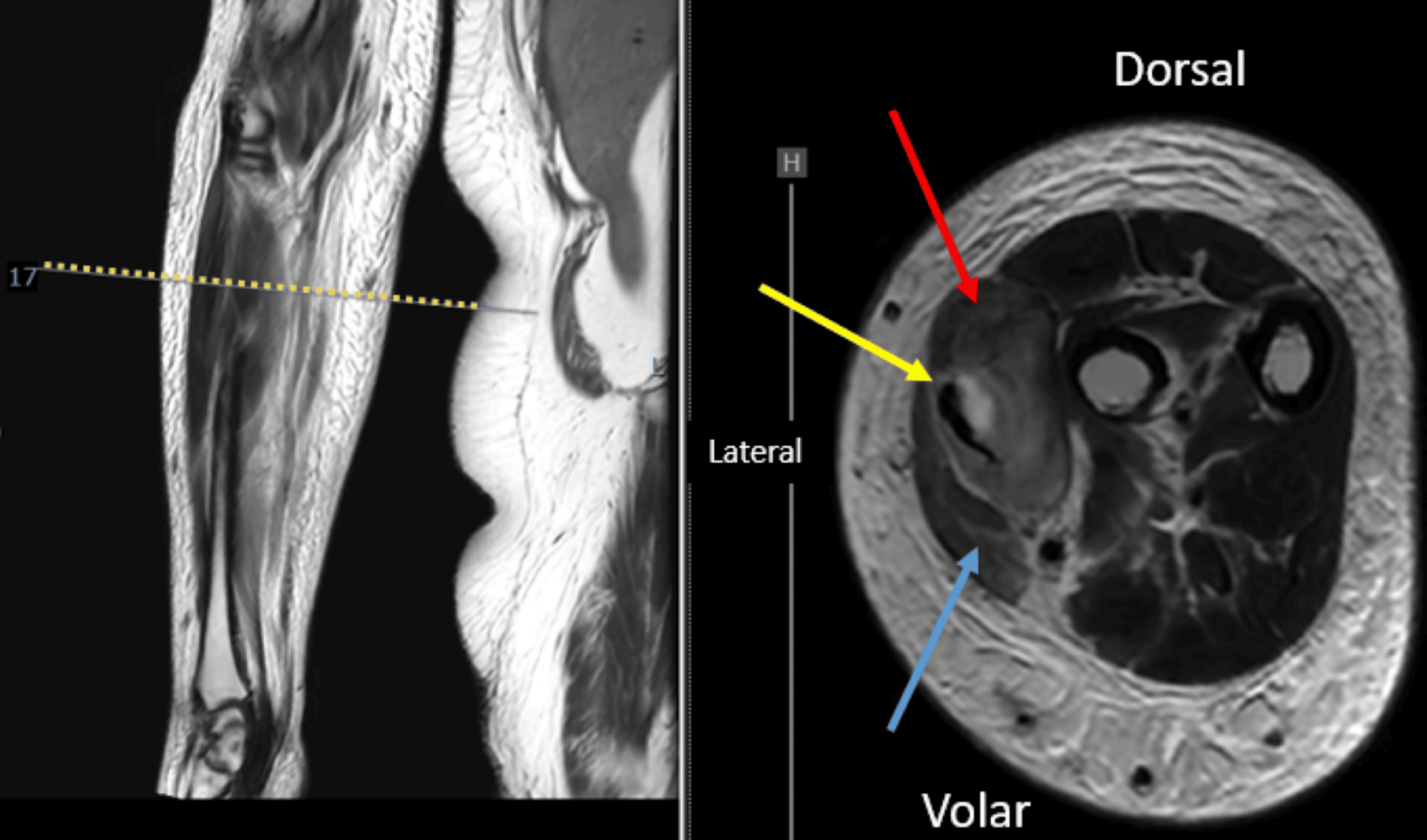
Magnetic resonance imaging The magnetic resonance imaging of the right forearm in Case 1 reveals an abscess within the lateral compartment. Red arrow, extensor carpi radialis muscles; blue arrow, brachioradialis muscle; yellow arrow, the abscess cavity
